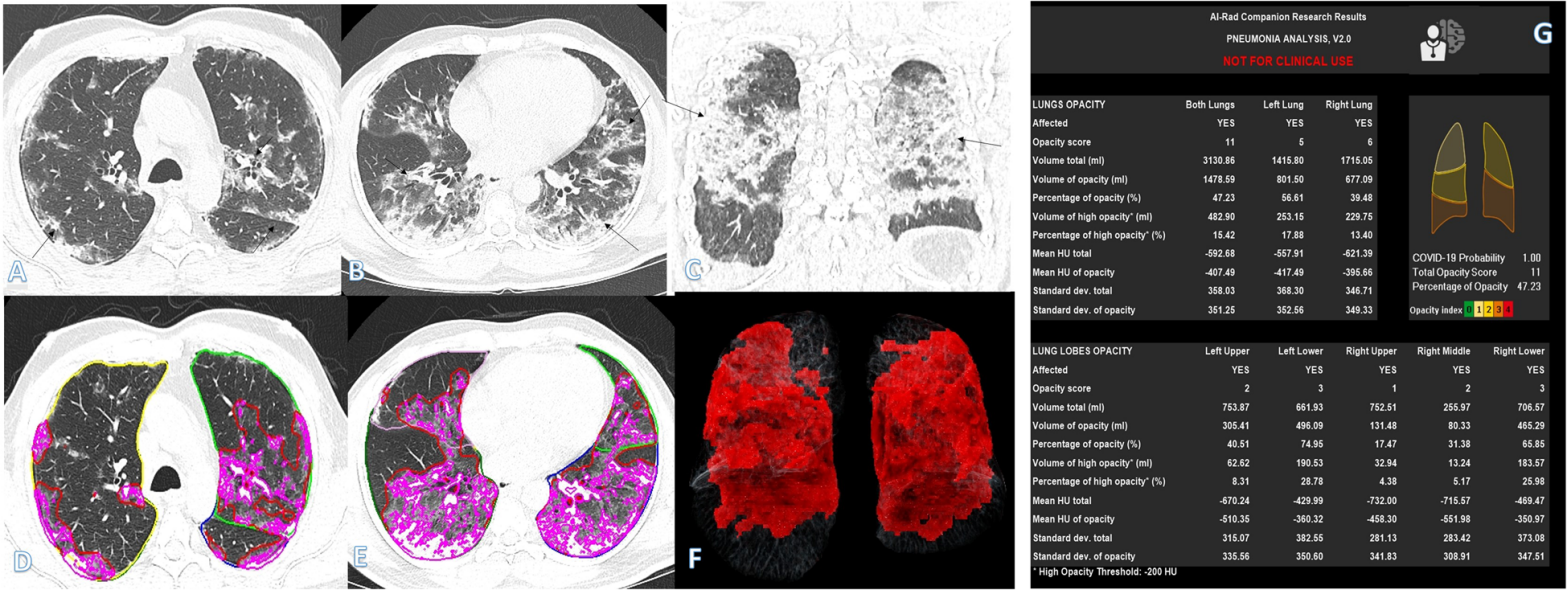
A 54-year-old male with positive COVID-19 virus. CT chest shows bilateral consolidation patches with air bronchogram, crazy-paving appearance mainly peripheral and basal, and subpleural lines (arrows) with more involvement of the left upper and both lower lobes (arrows in a–c). Total severity score = 11. Quantitative analysis (d–g) by AI-Rad Companion Research CT Pneumonia Analysis was presented with the measured parameters seen in table (g). Quantitative total opacity score was 11
